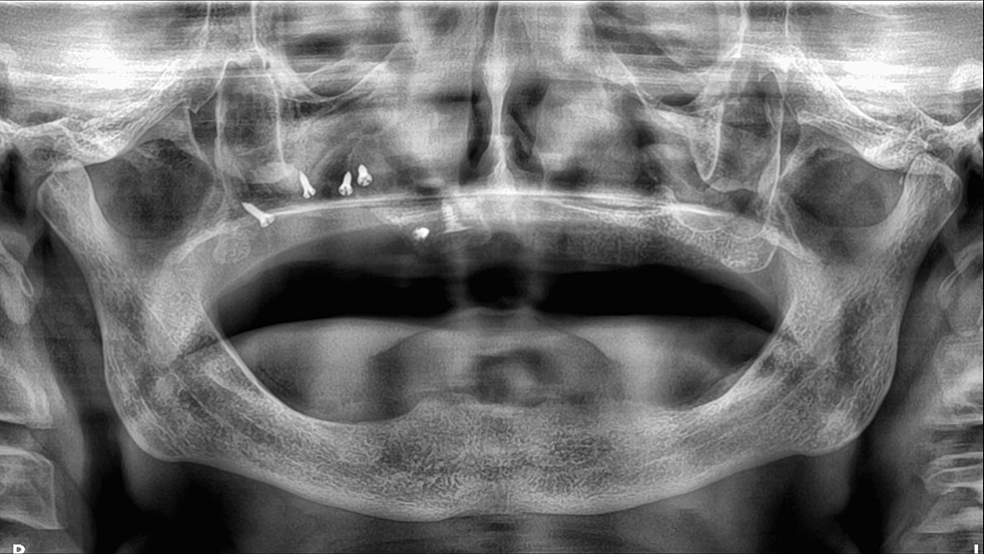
Immediate post-operative panoramic X-ray.
